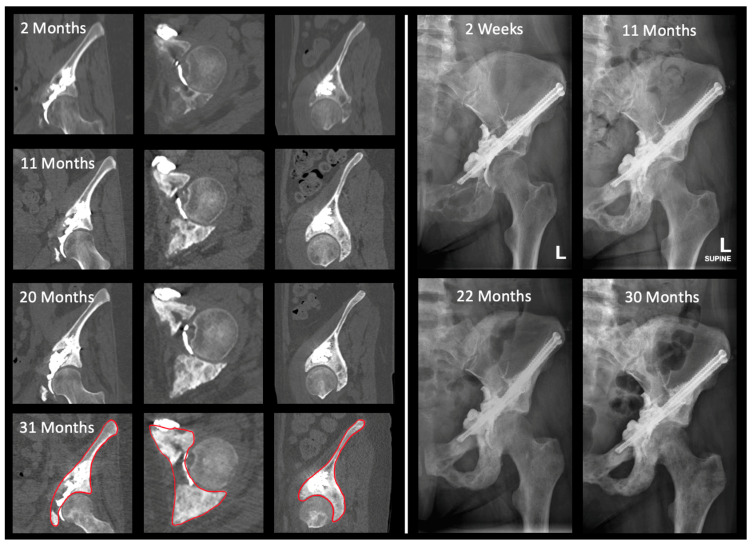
(Left panel) Progressive CT imaging showing coronal, axial, and sagittal regions of interest (femur was not included in region of interest selection). Red outline indicates the region of interest used in measurement. (Right panel) Progressive plain X-ray radiographs of similar time points. Throughout the post-operative period, the patient exhibited continual visceral organ cancer progression as well as documented skeletal disease progression in the pelvis, femur, sacrum, spine, and humerus. Patient received additional skeletal surgery in the humerus, contralateral acetabulum/femur region, and sacrum. Patient received 30 Gy in 10 fractions of radiation therapy, chemotherapy, and bisphosphonate regiments. Patient is alive and ambulating well at time of study censure. Measured bone mass change between 2 months and 31 months of 35.6% on 3D volumetric grayscale and 2D coronal, axial, and sagittal of 45.9%, 209.5%, and 34.6%, respectively.
